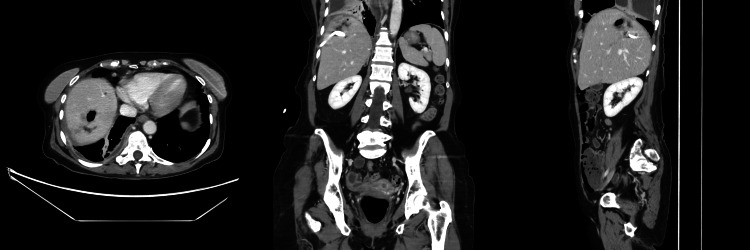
Follow-Up Computed Tomography (CT) at Two Weeks Post-Discharge. Follow-up computed tomography (CT) at two weeks post-discharge showed a reduction in abscess size within segment 8 of the liver, now measuring 3.5 cm craniocaudal (previously 8.9 cm) and 3.1 cm in axial diameter (previously 8.1 cm). The drainage catheter remained in place. No new hepatic lesions were identified. The catheter was subsequently removed without complication.
